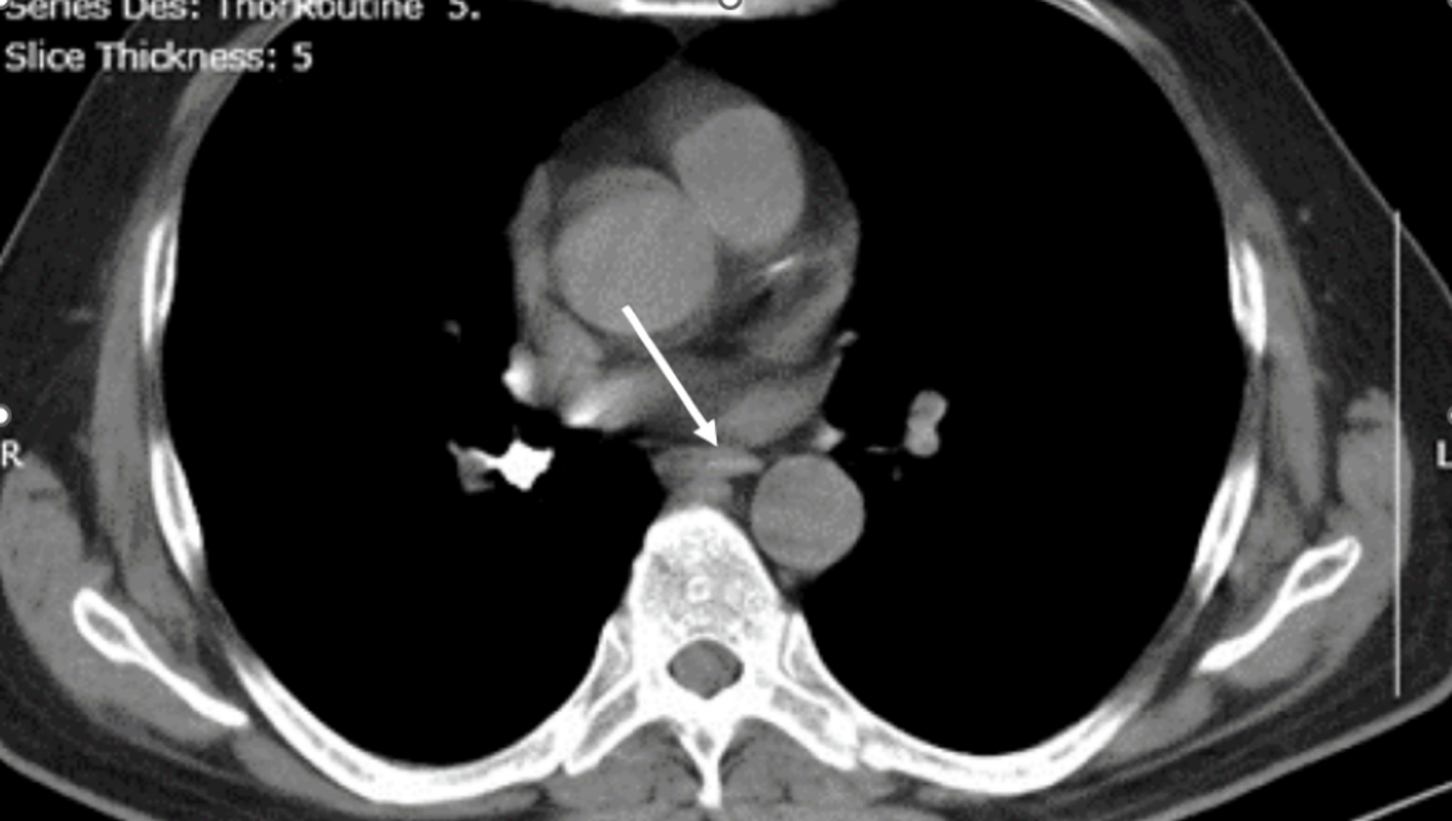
CT scan showing the impaction of the implant driver in the right main inferior bronchus.
